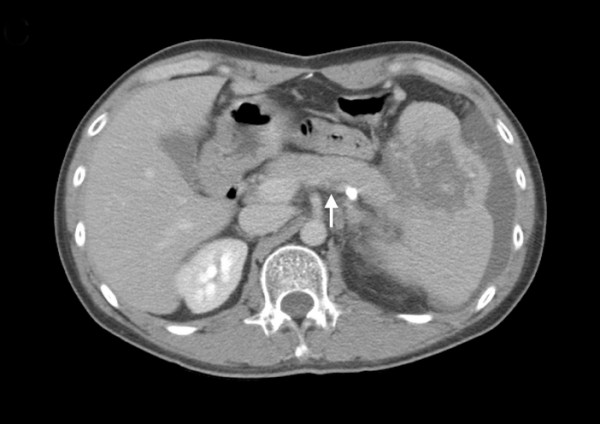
Abdominal computed tomography scan showed a filling defect in the splenic vein (arrow), indicating thrombosis formation.
